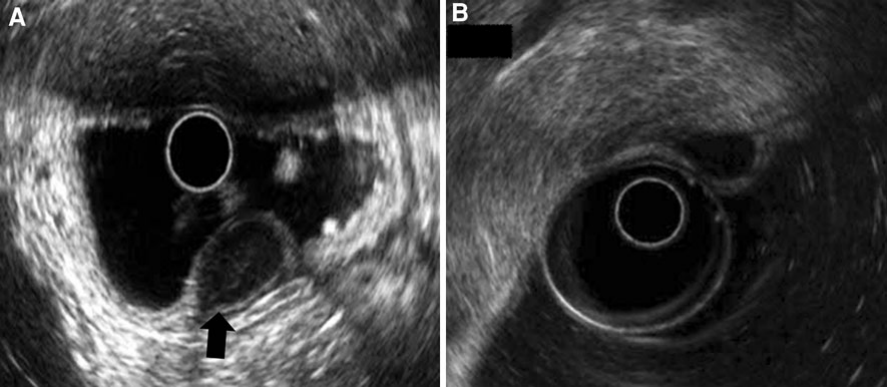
The degree of muscular connection with a subepithelial tumor as shown by endoscopic ultrasound. A Narrow muscular connection with the tumor: the diameter of the connection with the fourth layer of the tumor is <50 % of the maximal diameter of the tumor base (*black arrow* hyperechoic demarcation line between the tumor and the muscularis propria layer). B Wide muscular connection with the tumor: the diameter of the connection with the fourth layer is >50 % of the maximal diameter of the tumor base
